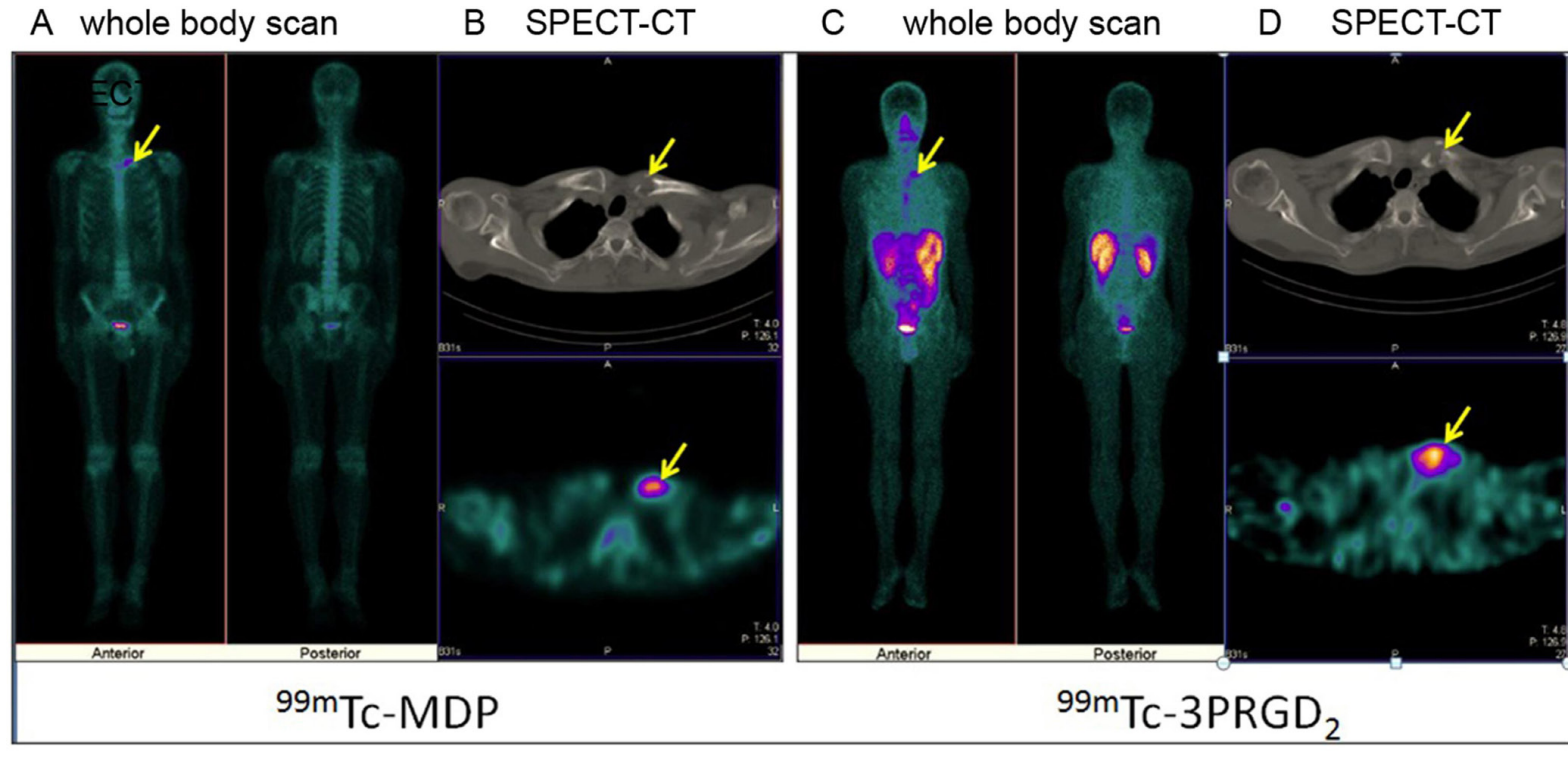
^99m^Tc-MDP imaging versus ^99m^Tc-3P-RGD_2_ imaging [Whole Body Scan (WBS) and SPECT-CT) in a patient with lung cancer Osteolytic bone metastases in the left clavicular head demonstrates as “hot region” on both ^99m^Tc-MDP A., B. and ^99m^Tc-3P-RGD_2_ imaging C., D. and bone destruction on CT(B,D) . A: ^99m^Tc-MDP WBS; B: ^99m^Tc-MDP SPECT-CT; C: ^99m^Tc-3P-RGD_2_ WBS; B: ^99m^Tc-3P-RGD_2_ SPECT-CT. (see arrows).
